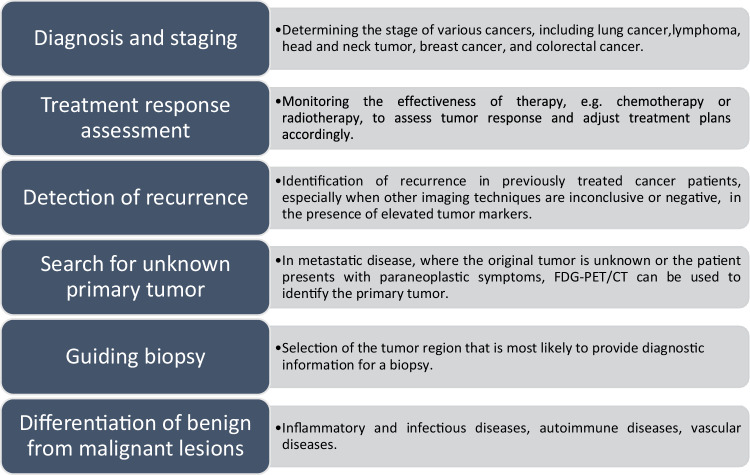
Clinical indications of [^18^F]FDG-PET/CT in oncology
